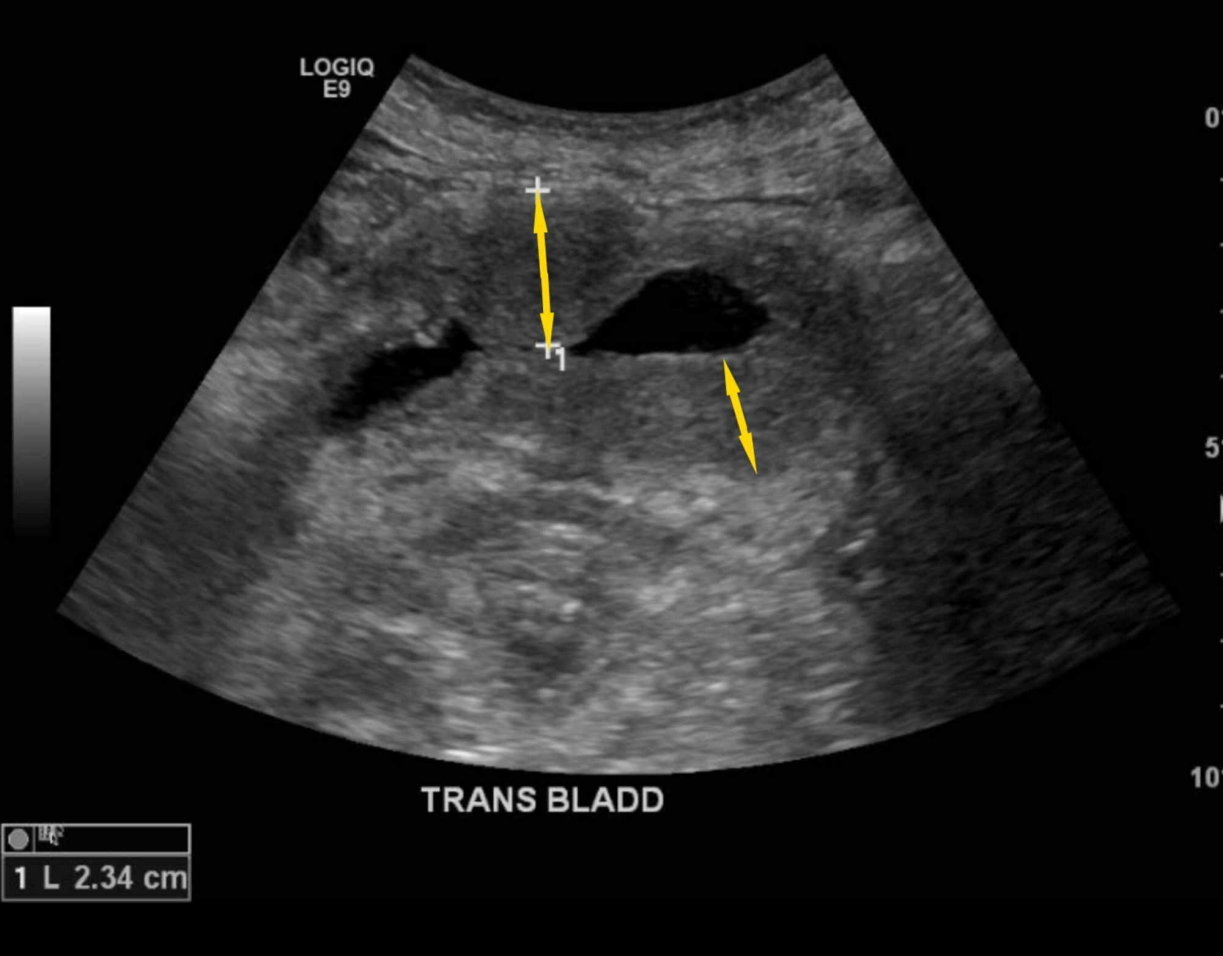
Ultrasound picture showing the bladder wall thickening
